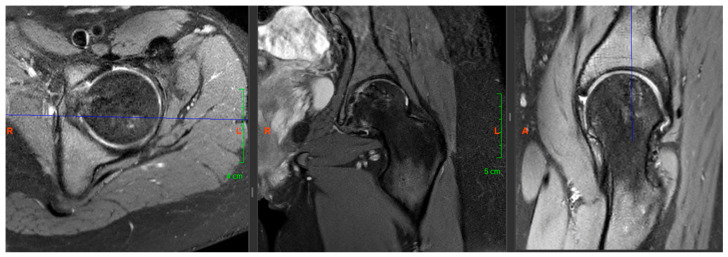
Postoperative MRI scan taken at 3 years of age, showing good joint congruence and proper integration of the grafts.
